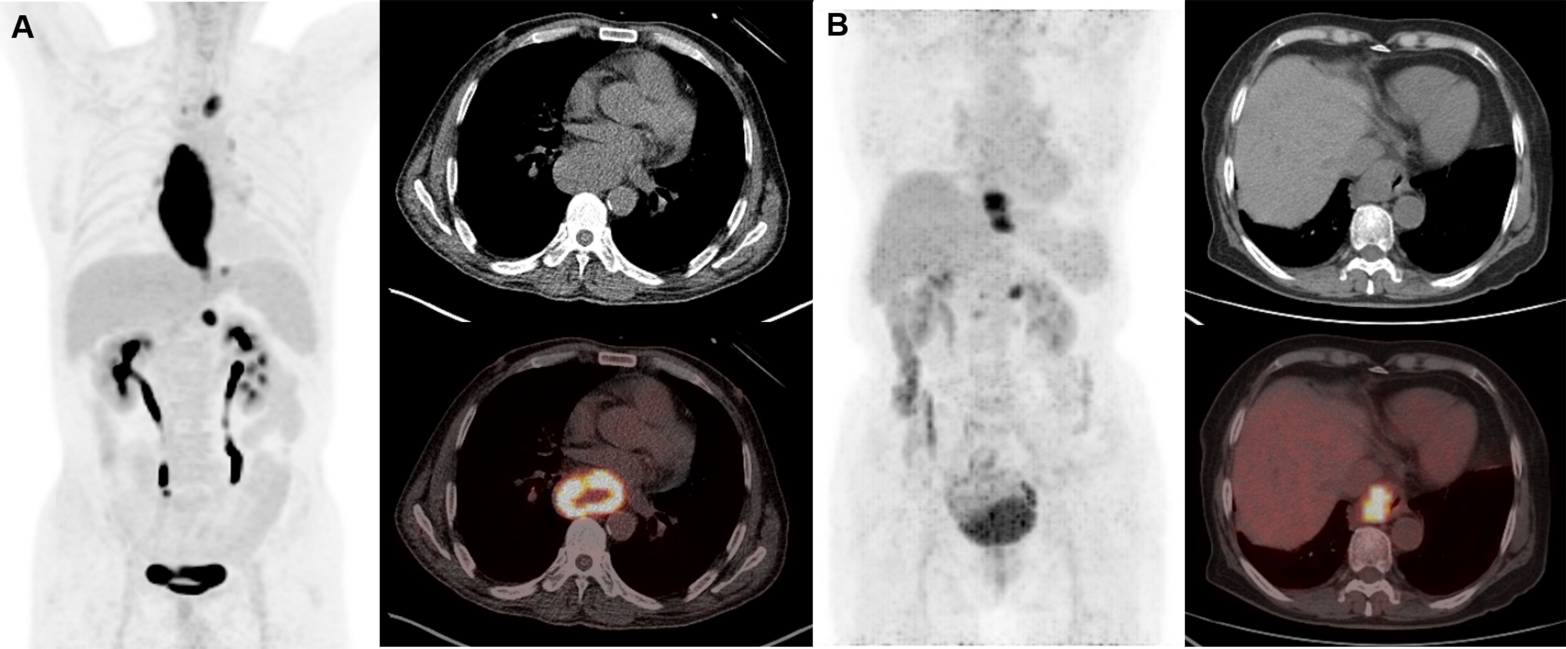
Representative cases of metabolic parameters affecting prognosis. Patient A was a 60-year-old man (SUVmax 23.9, SUVmean 8.8, TBR 16.0, TLR 11.3, MTV 213, TLG 1874.7). He had lymph node metastases at initial diagnosis and received chemotherapy. This patient suffered from lung recurrence and died from the disease at 12 months after initial treatment. Patient B is a 76-year-old woman (SUVmax 8.7, SUVmean 4.6, TBR 6.4, TLR 4.5, MTV 22.9, TLG 104.9). She had lymph node metastases at initial diagnosis and received radiotherapy and chemotherapy. This patient remains progression free for 96 months and is still alive until the last follow-up. Compliance with ethical standards.
